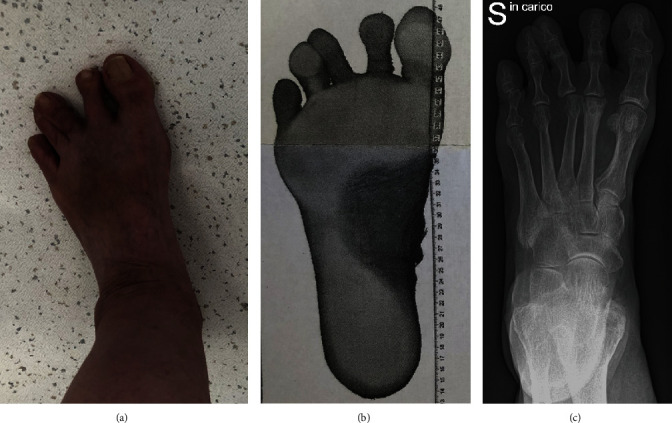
(a) Splay toe between 2^nd^ and 3^rd^ toe. (b) Dynamic analysis of the foot while weight bearing. (c) Plane X-ray of the foot showing Smillie stage V necrosis of the 2^nd^ metatarsal head causing the splay toe deformity with a relatively long 3^rd^ metatarsus.
